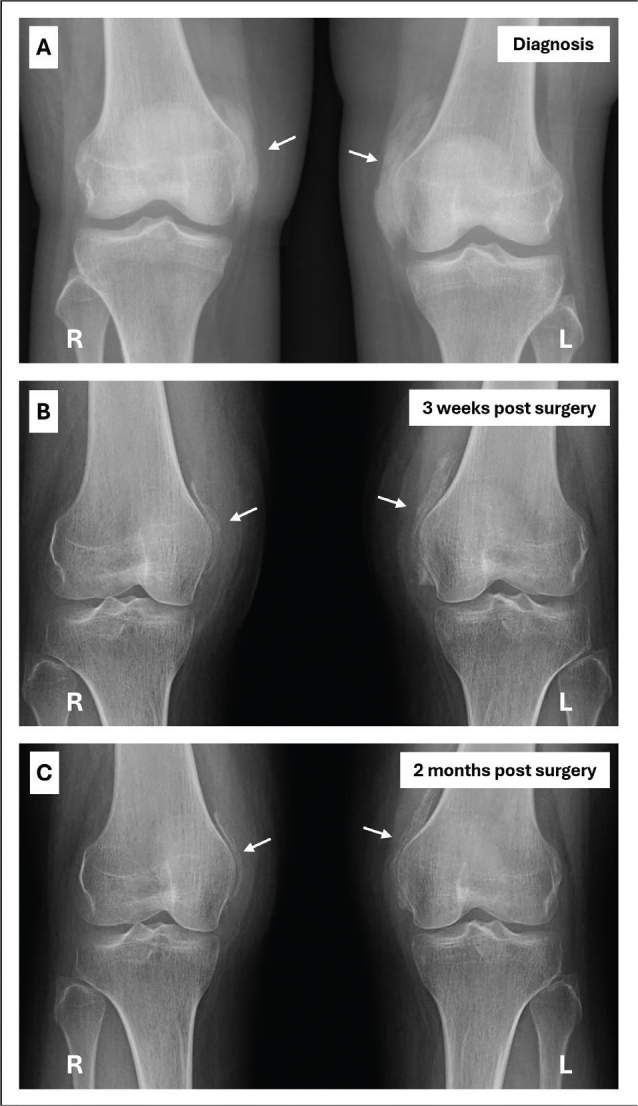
Sequential anteroposterior radiographical images showing bilateral extra-articular calcifications on the anteromedial side of the knees (white arrows). (A) Radiological diagnosis of heterotopic ossification. (B) Three weeks after surgery. (C) Two months after surgery.
